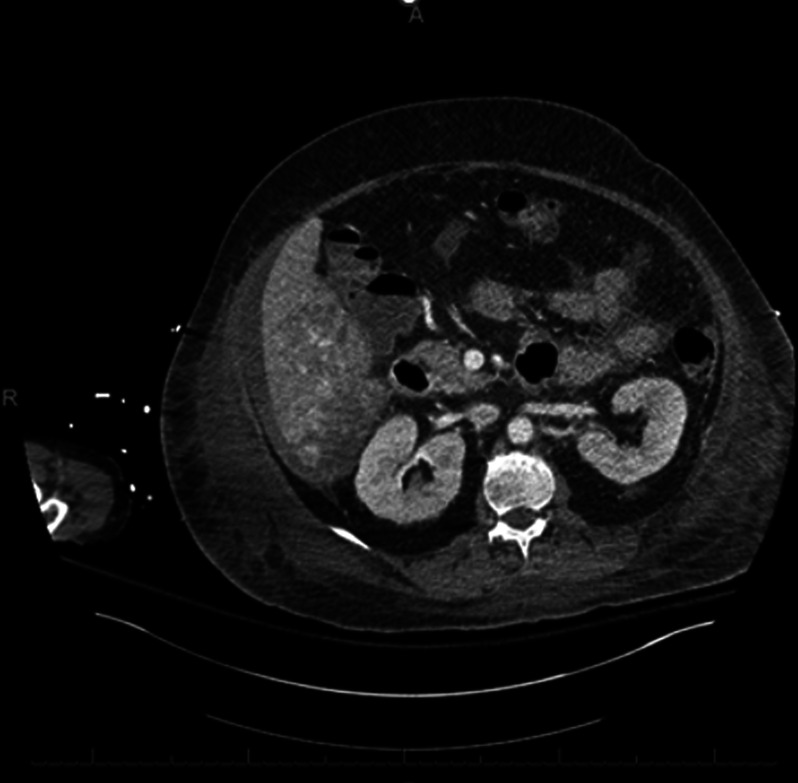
Abdominal computed tomography (3 days after admission) showing linear area of hypoattenuation within the inferior aspect of the right hepatic lobe extending to the periphery correlating to an appearance seen with laceration.
